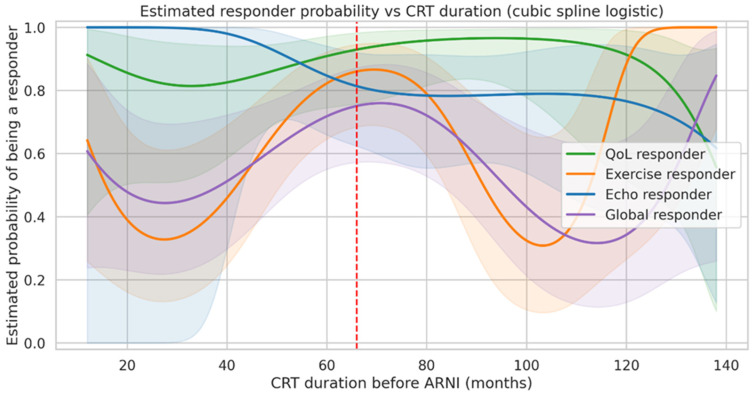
Estimated probability of treatment response according to CRT duration. The probability of being classified as a responder is shown as a function of CRT duration prior to ARNI initiation, modeled using logistic regression with cubic spline functions. Separate curves are displayed for QoL, exercise, echocardiographic, and global response. Shaded areas represent 95% confidence intervals. The dashed vertical line indicates the approximate transition zone around 66 months (5.5 years). Predictions are displayed only within the range of CRT duration supported by the observed data.
